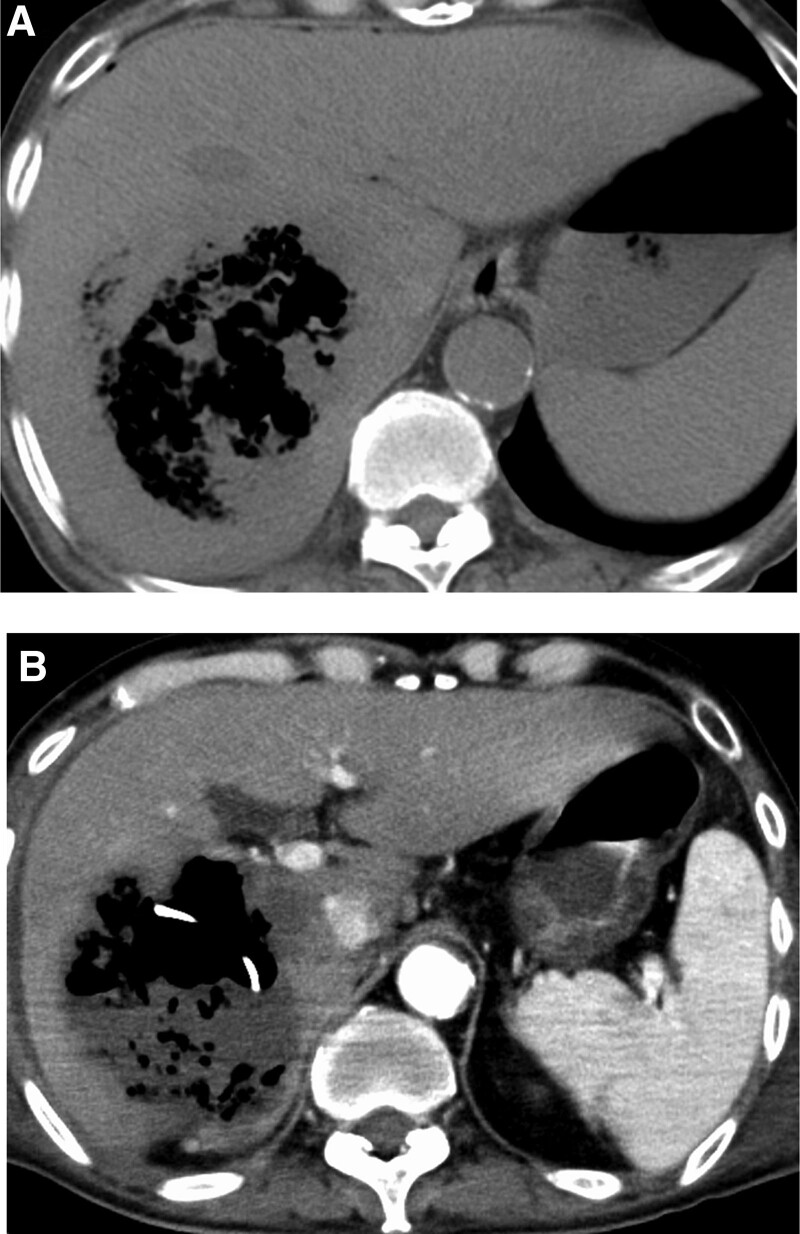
Abdominal computed tomography showing a gas-forming pyogenic liver abscess after atezolizumab plus bevacizumab treatment and (A) before and (B) after percutaneous liver abscess drainage.
